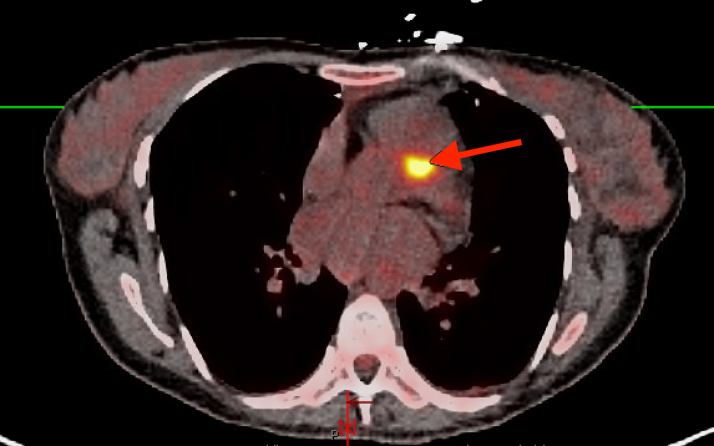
Positron Emission Tomography/Computed Tomography Visualization of Hypermetabolic Intracardiac Lesion Positron emission tomography/computed tomography showing area of increased metabolic activity along anterior aortic root (arrow).
